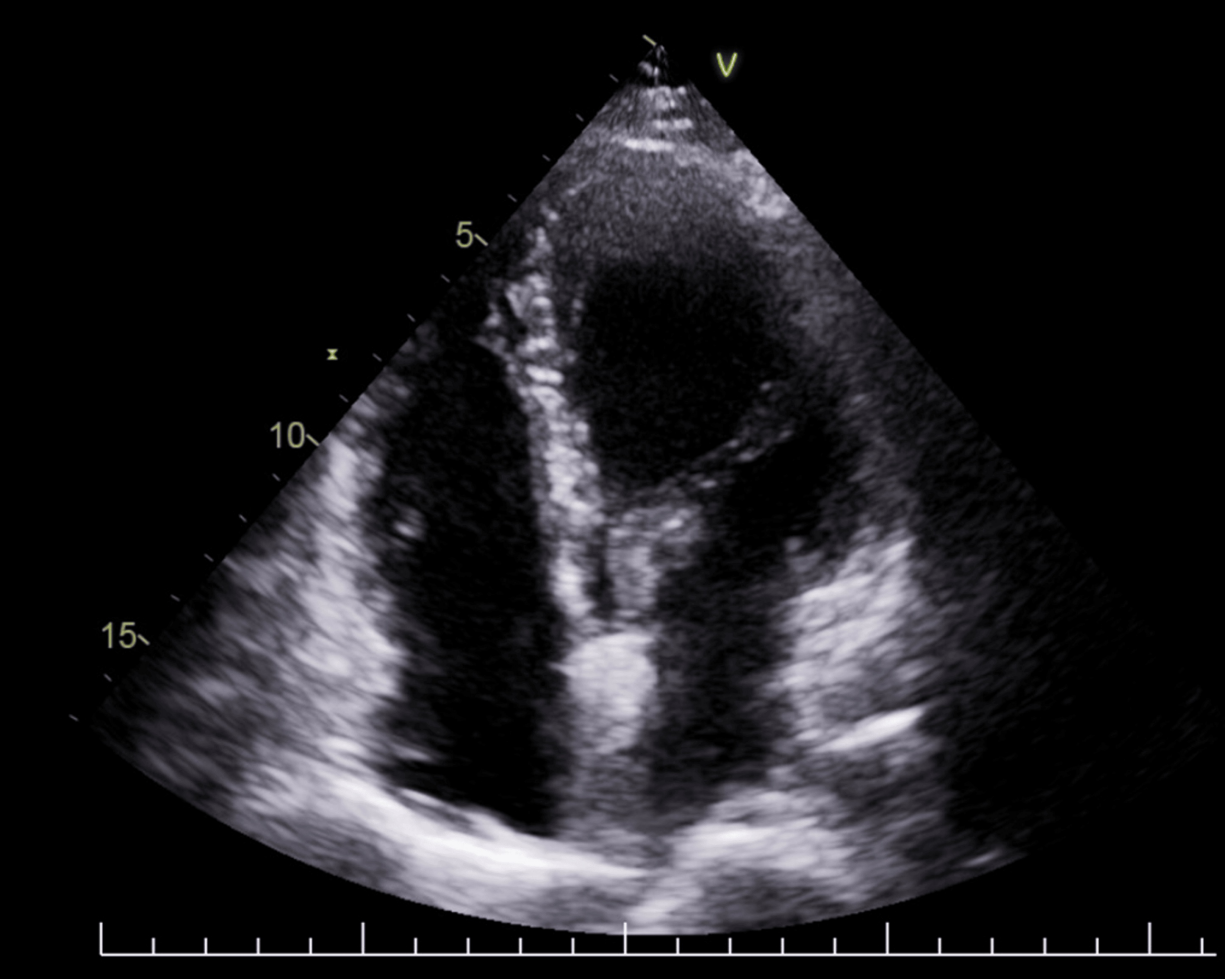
Repeat echocardiogram at 3 months post-discharge showing complete resolution of right atrial and right ventricular thrombi
